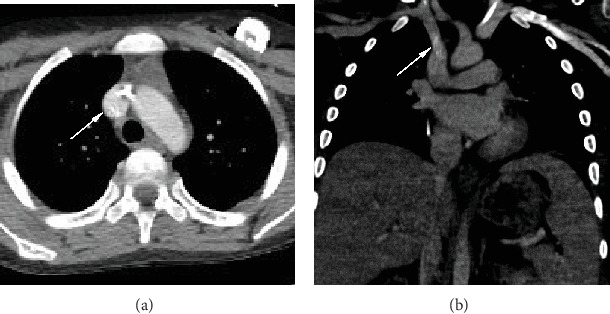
Chest CT ordered to evaluate for pulmonary fungal disease revealed proper placement of a left-sided chest port and a calcified tubular structure (arrows) representing a calcified CRS near the junction of the right brachiocephalic vein and superior vena cava on axial (a) and coronal (b) views.
